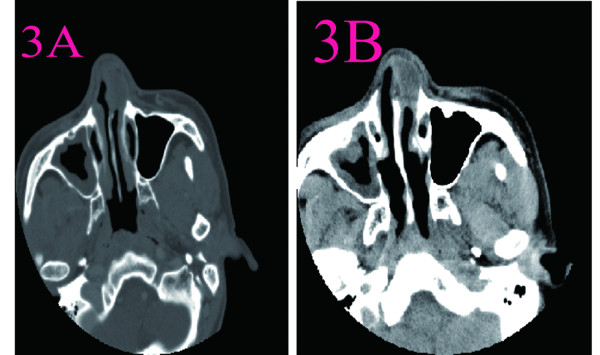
CT scans of nasal cavity in Case 2.A: CT imaging showed that a well-defined round soft tissue mass without central in the right nasal vestibule. The mass of density was uneven(20 ~ 36Hu). B: There was patchy enhancement on contrast CT.
